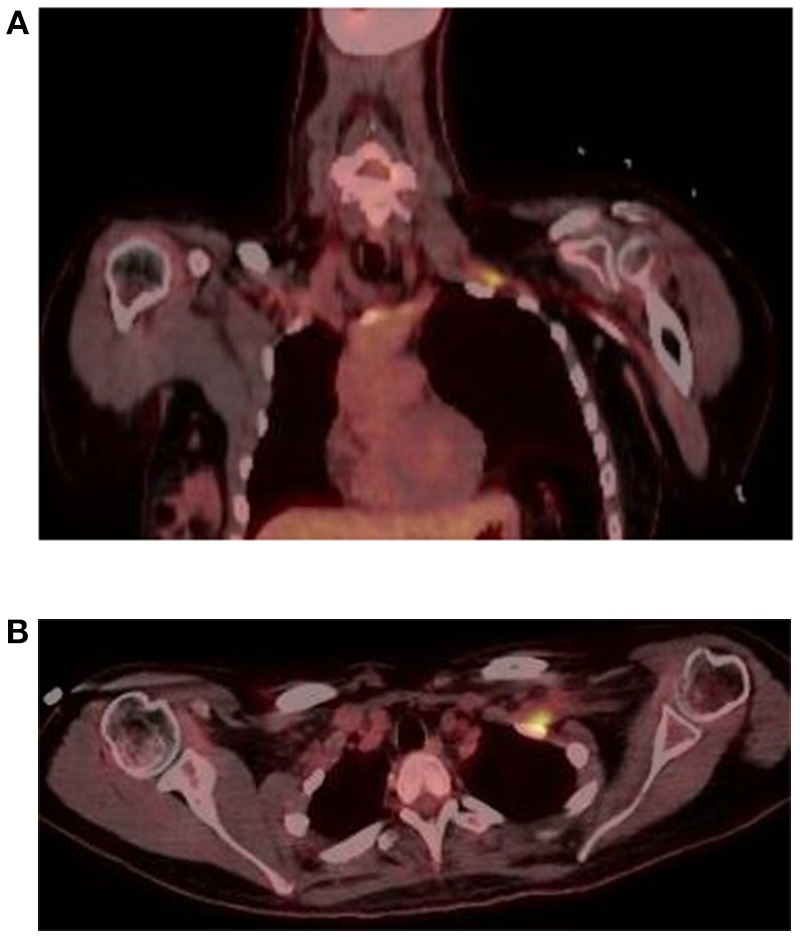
FDG PET coronal (A) and axial (B) sequences show focal hyperactivity at the left pectoral/subpectoral level adjacent to the first rib.
